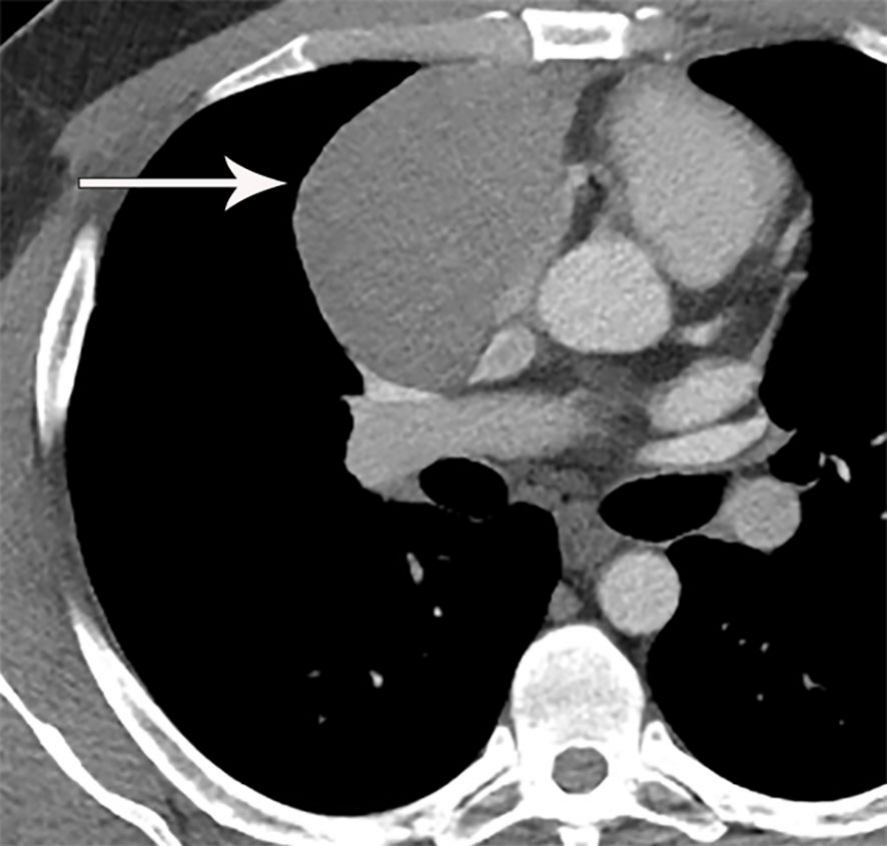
32 year old man with thymoma and myasthenia gravis. Contrast-enhanced CT shows right prevascular mediastinal mass (arrow).
